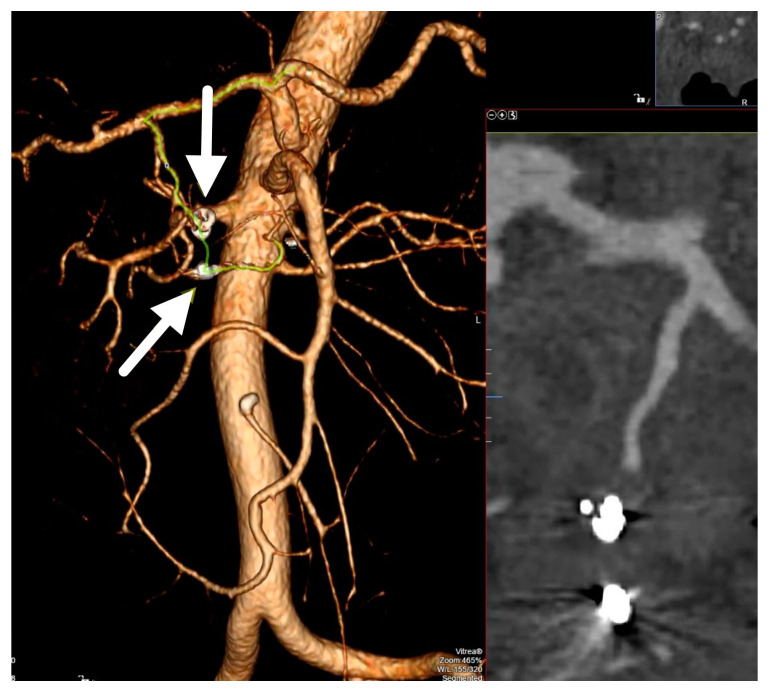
3D volume rendering CT image shows the complete exclusion of the pseudoaneurysm with coils.
